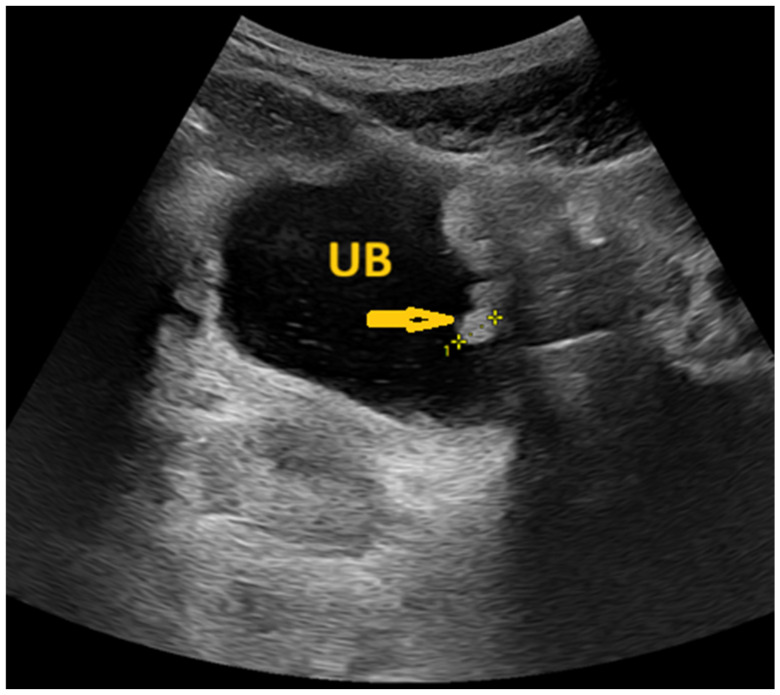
Selected ultrasonography image of a 20-year-old male patient shows multiple polypoidal thickening in the left urinary bladder wall, the largest measuring 10 × 5 mm (arrow), reflecting *Schistosoma* cystitis. UB: Urinary bladder.
